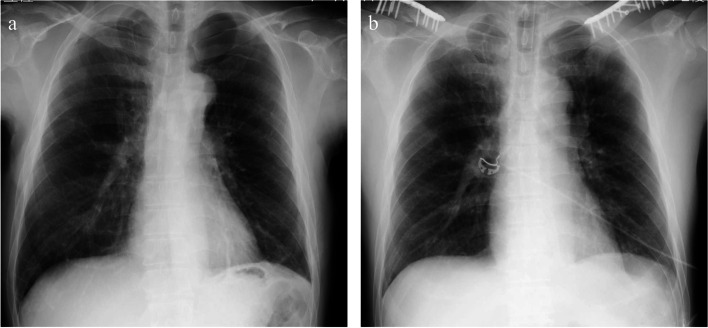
a Chest radiograph image was taken 2 days after being injured, revealing bilateral midshaft clavicle fractures and left pneumothorax. b Chest radiograph image taken immediately after surgery, showing bilateral repaired clavicle fractures
